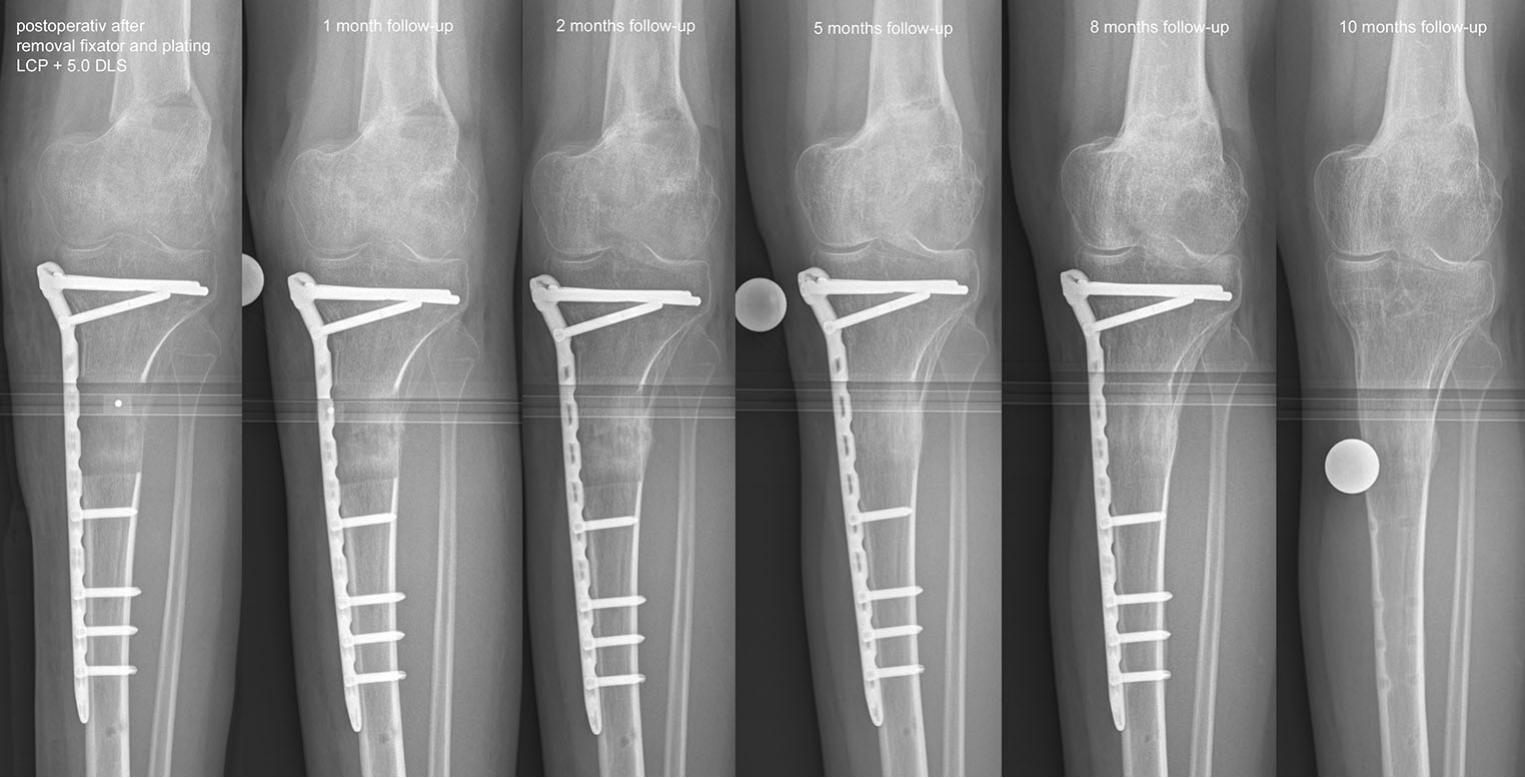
Follow-up radiographs. Osteosynthesis with a nine-hole LCP in conjunction with three DLS 5.0 proximal and four LS 5.0 distal. Continuous bone healing. After 2 months, criteria for a biomechanically stable situation are visible. After 5 months, complete homogenous bony structures are visible
